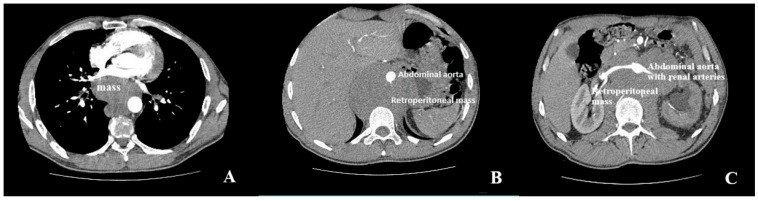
Contrast-enhanced computed tomography showing thoracic and retroperitoneal masses. (A) Axial contrast-enhanced CT showing a posterior mediastinal mass adjacent to the heart. (B) Axial abdominal CT showing a bulky retroperitoneal mass encasing the abdominal aorta, resulting in the “floating aorta” sign. (C) Axial abdominal CT showing a retroperitoneal mass encasing the abdominal aorta and renal vessels.
